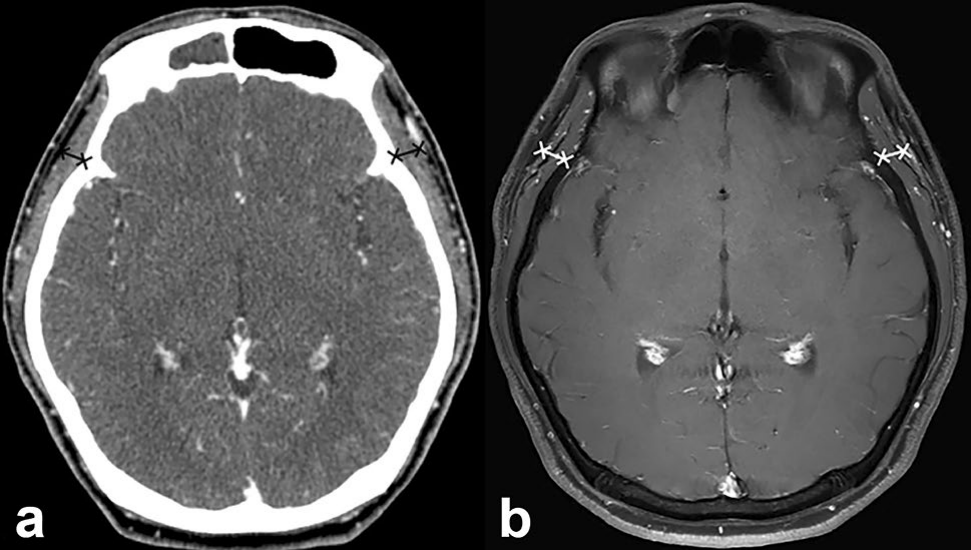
Representative case for the assessment of temporalis muscle thickness (TMT) on CT and MRI. A 41-year-old patient has an averaged TMT of 6.66 mm on CT (a) and 8.04 mm on MRI (b).
